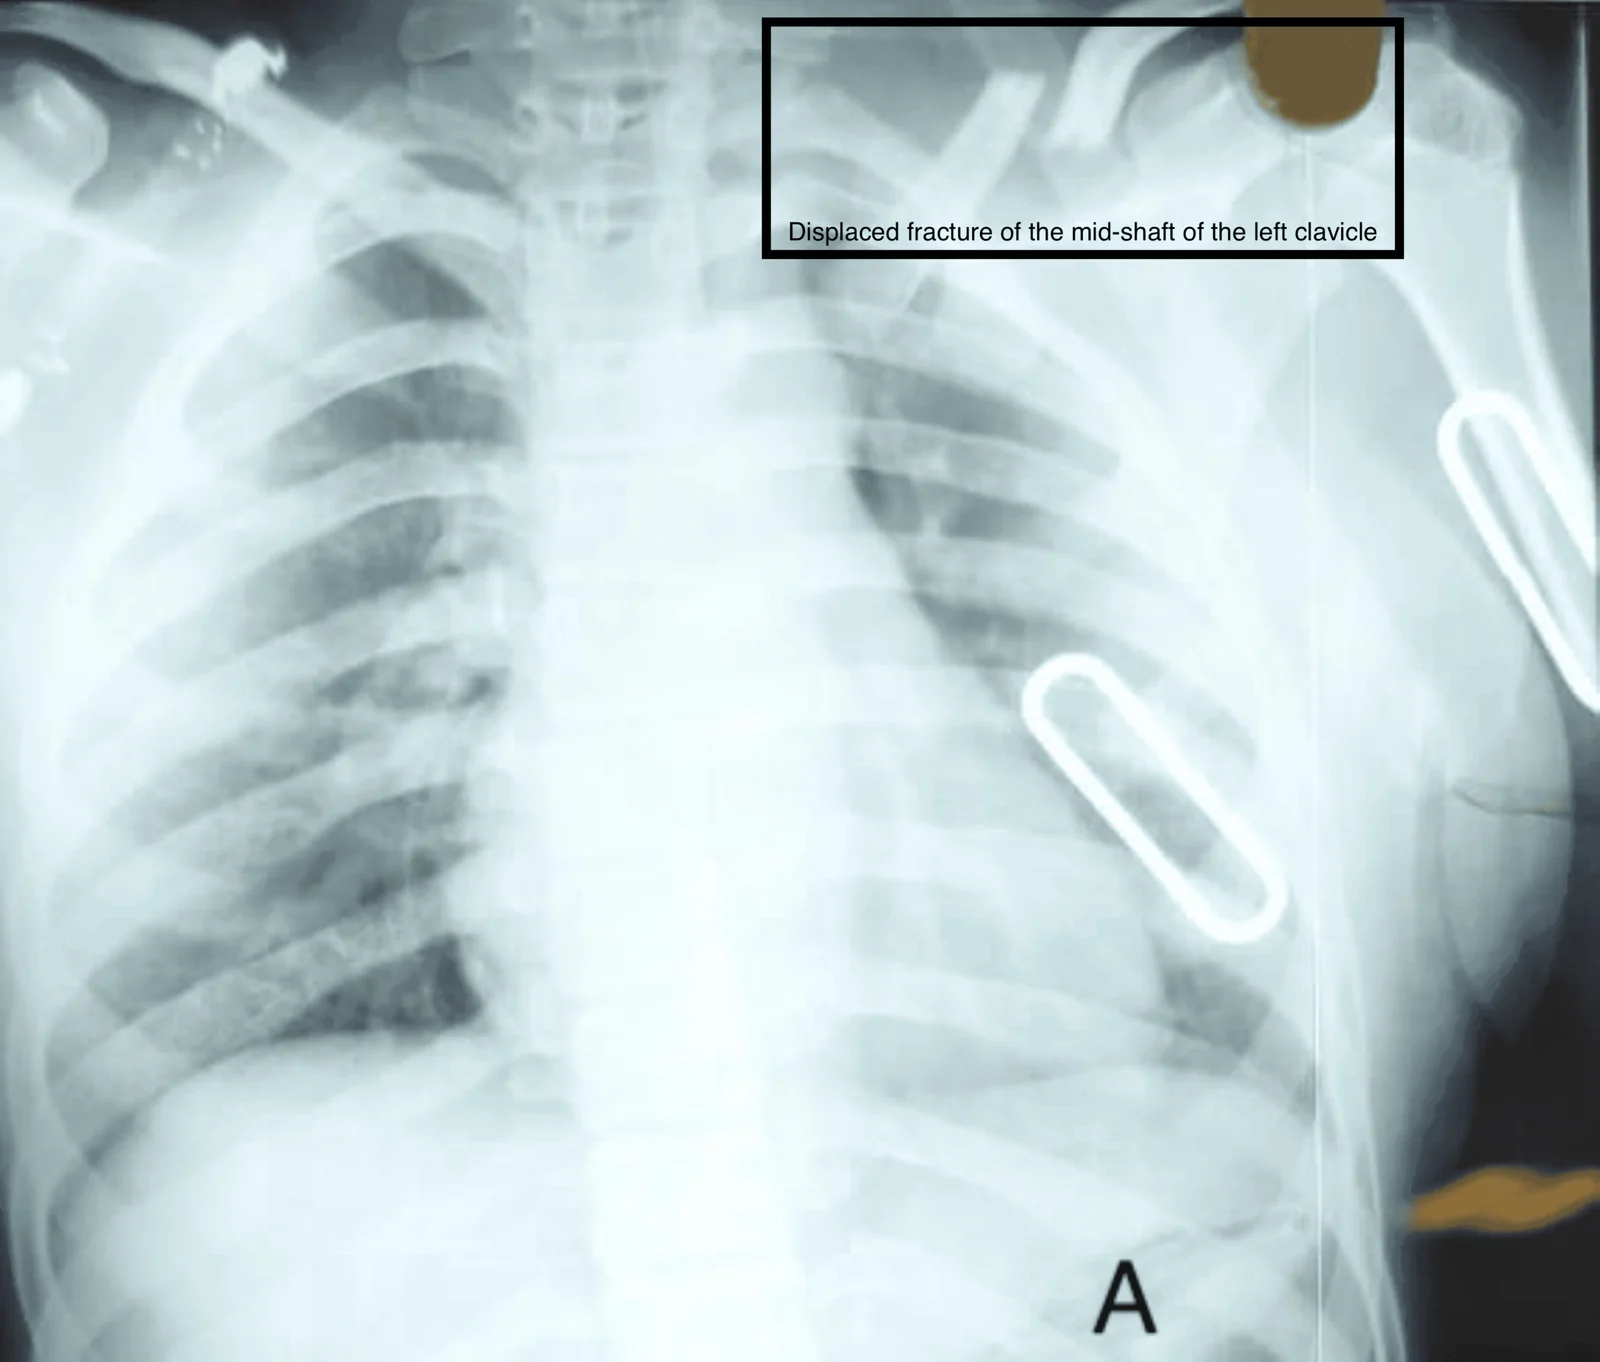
AP chest radiograph of an 18-year-old girl showing a displaced fracture of the mid-shaft of the left clavicle. AP: Anteroposterior
